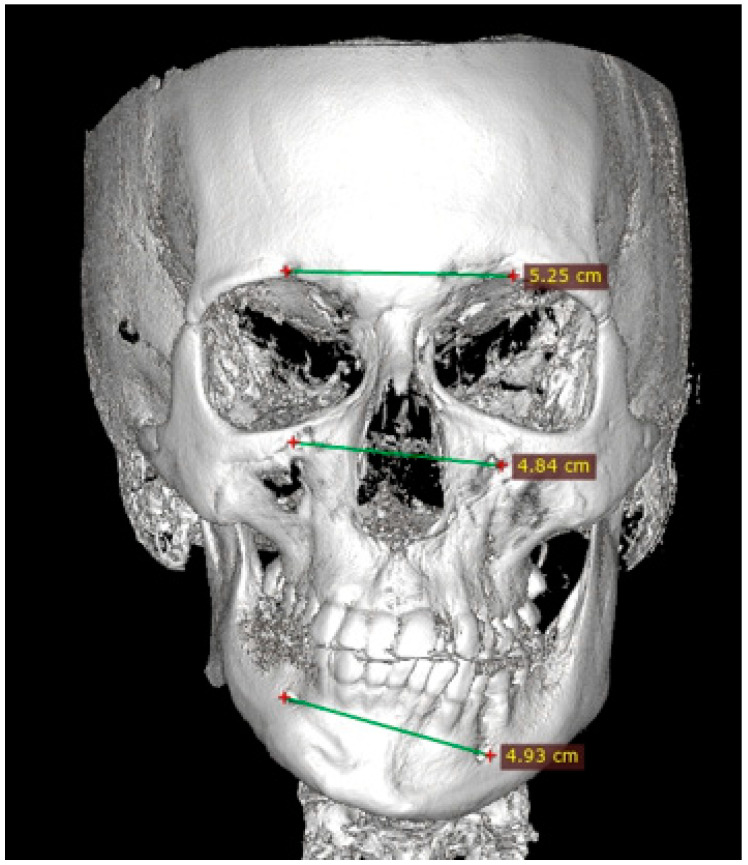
CBCT measurements in maxillo-mandibular asymmetry caused by condylar hyperplasia—from top the SOF, IOF, and MFO measurements in horizontal aspect. Additional horizontal lines indicate the foramina tilting and scope of asymmetry compared to the opposite healthy side and the skeletal midline.
